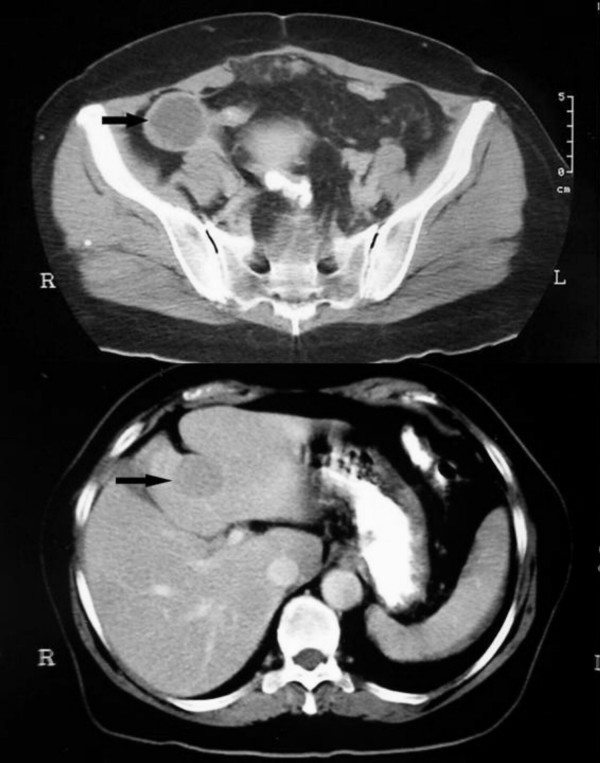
The initial CT scan demonstrates a mass in the right iliac fossa (arrow) and a suspected metasasis in segment IV of the liver.
